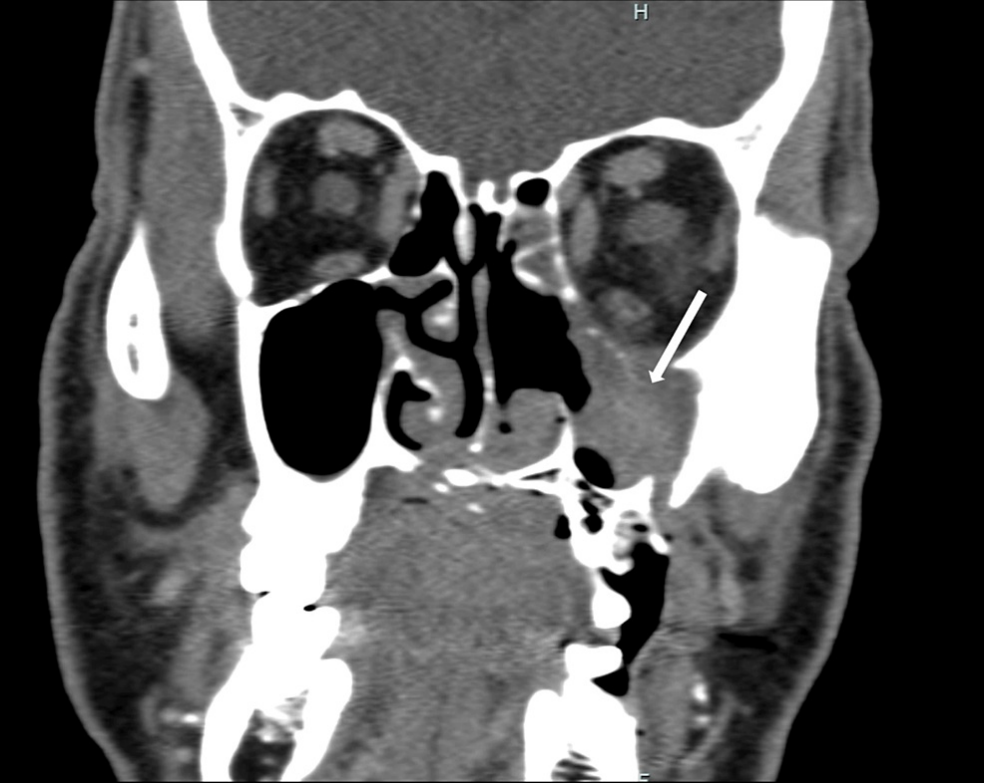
Contrast-enhanced computed tomography (CT) scan of the head and neck. The white arrow indicates the fungal infiltration of the left extraconal space.
